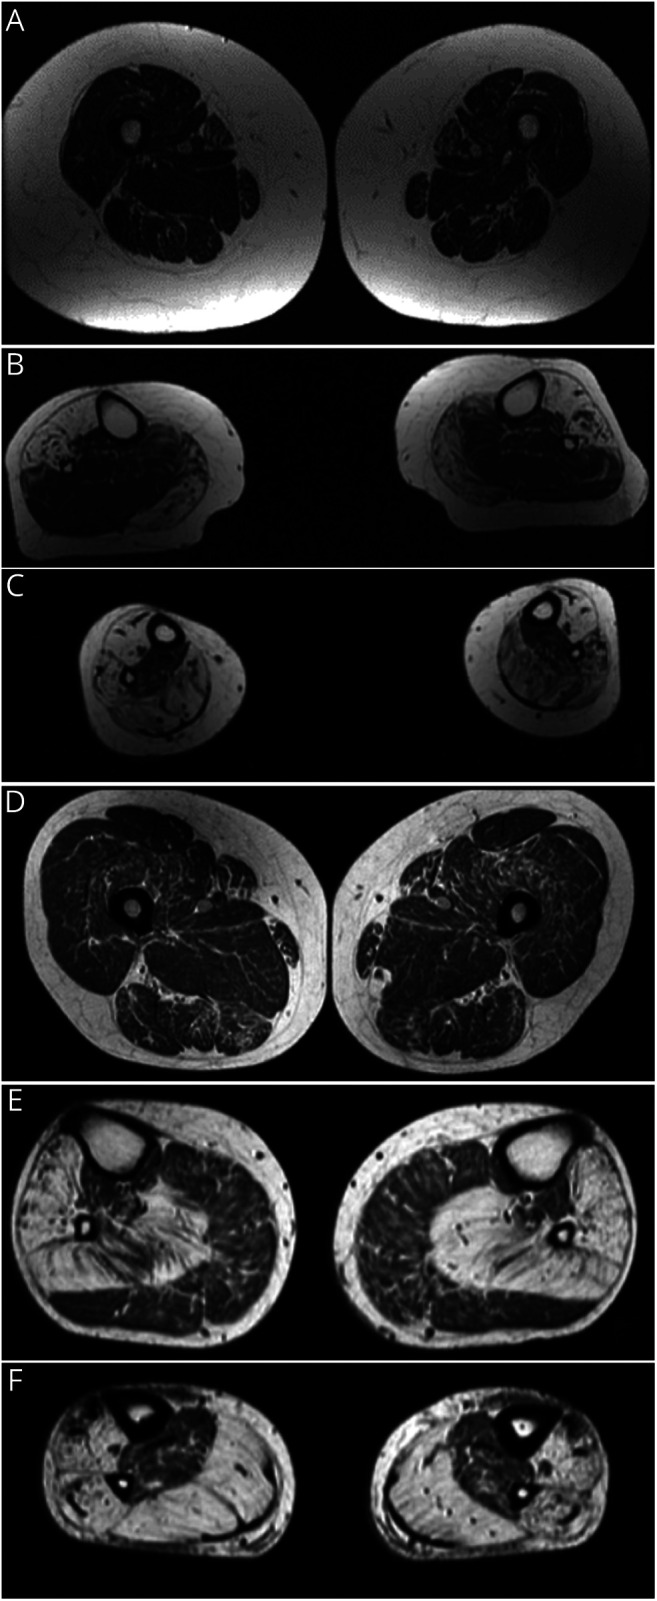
Muscle MRI Findings in the Finnish (A–C) and Italian (D–F) Probands (A) Thigh: normal; (B and C) lower leg: anterolateral compartment muscles, gastrocnemius medialis, and distal soleus muscles bilaterally are replaced by fibrofatty tissue. (D) Thigh: mild diffuse fatty degenerative changes in vastus intermedius and hamstring muscles; (E and F) lower leg: anterolateral compartment muscles and bilateral soleus muscles are completely replaced by fibrofatty tissue. Mild changes in gastrocnemius medialis.
